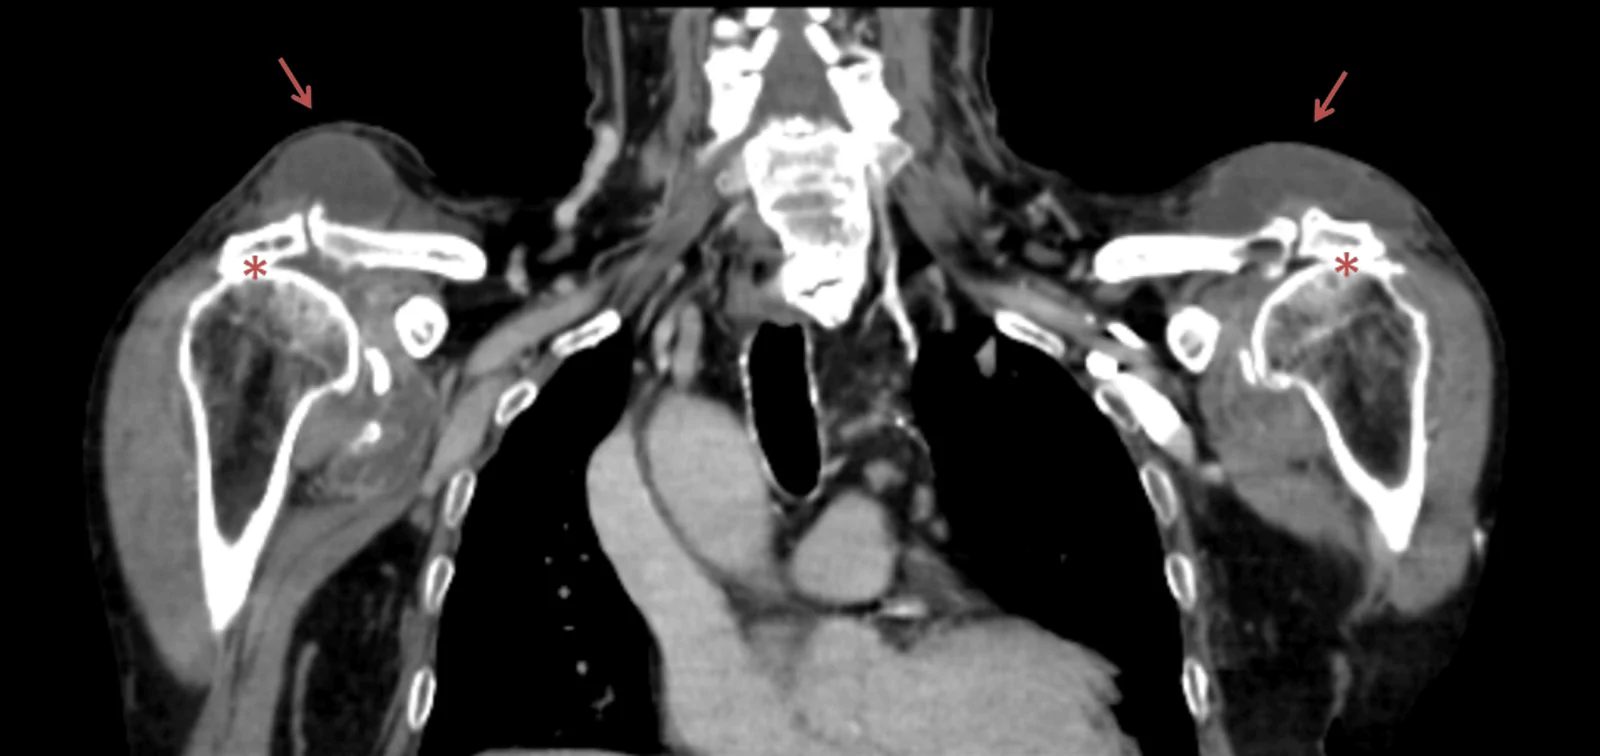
Coronal CT image of the shoulders demonstrating bilateral ACJ cysts (arrows) and imaging features suggestive of chronic rotator cuff tears (asterisks), with associated degenerative shoulder arthropathy, consistent with the GS. ACJ, acromioclavicular joint; GS, Geyser Sign
